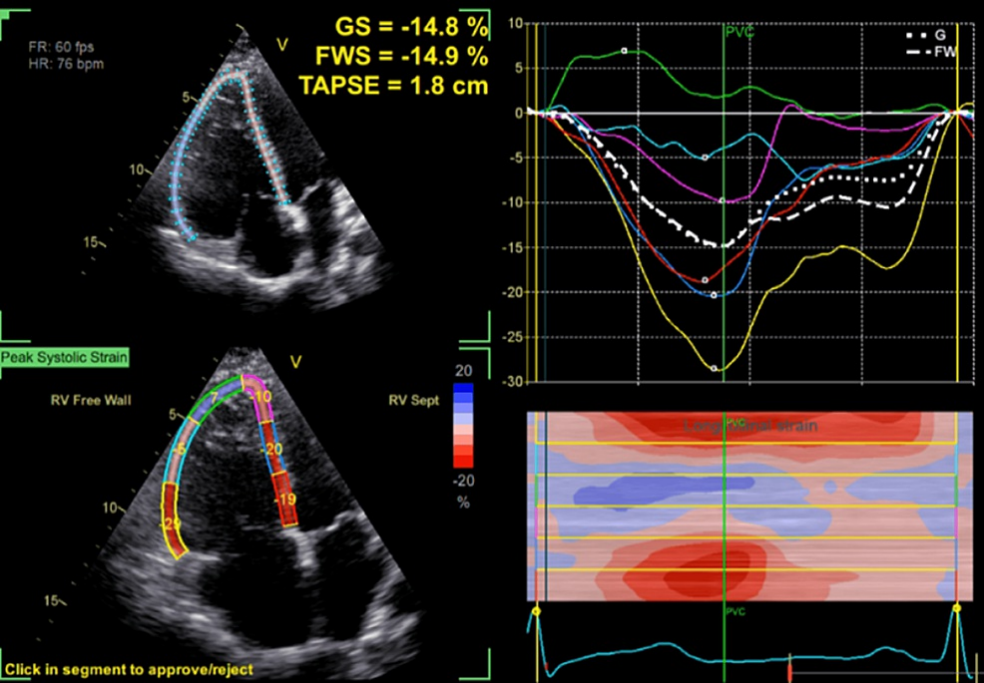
Speckle tracking echocardiography in modified apical four-chamber view showing abnormal right ventricular free wall and global strain values at -15%, with evidence of dyskinesia at the RV apex and positive regional strain of 7%. GS: global longitudinal strain; FWS: free wall strain; TAPSE: tricuspid annular plane systolic excursion; RV: right ventricle
